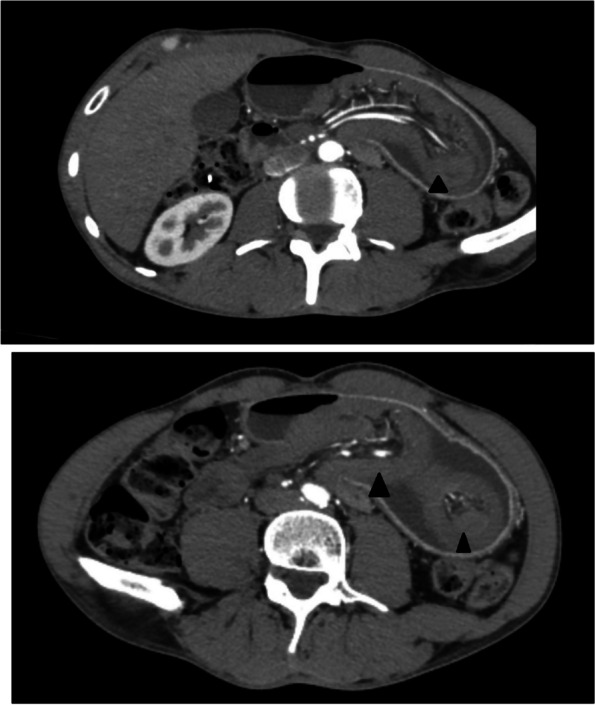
Enhanced CT imaging showing jejunal loops inside the stomach (white arrows)
